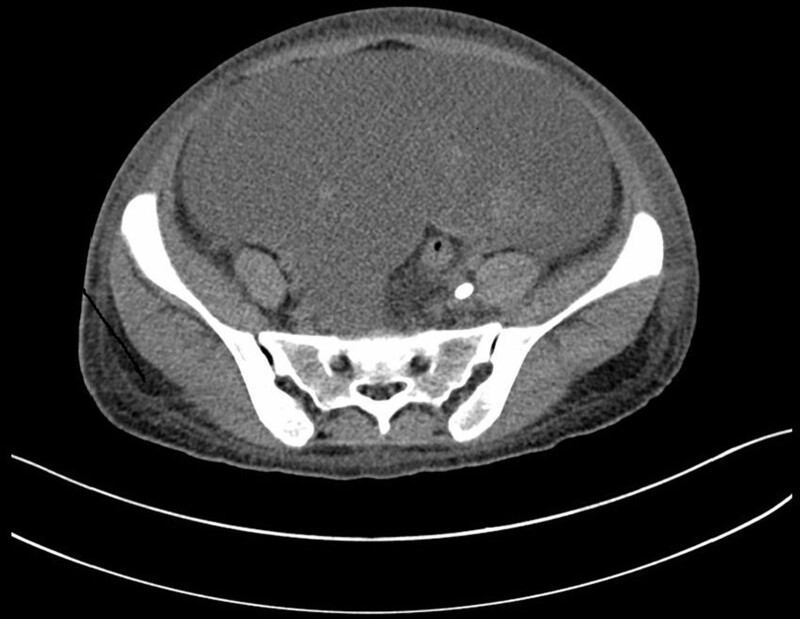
Abdominal and pelvic computed tomography angiography showing no obvious signs of vascular hemorrhage.
